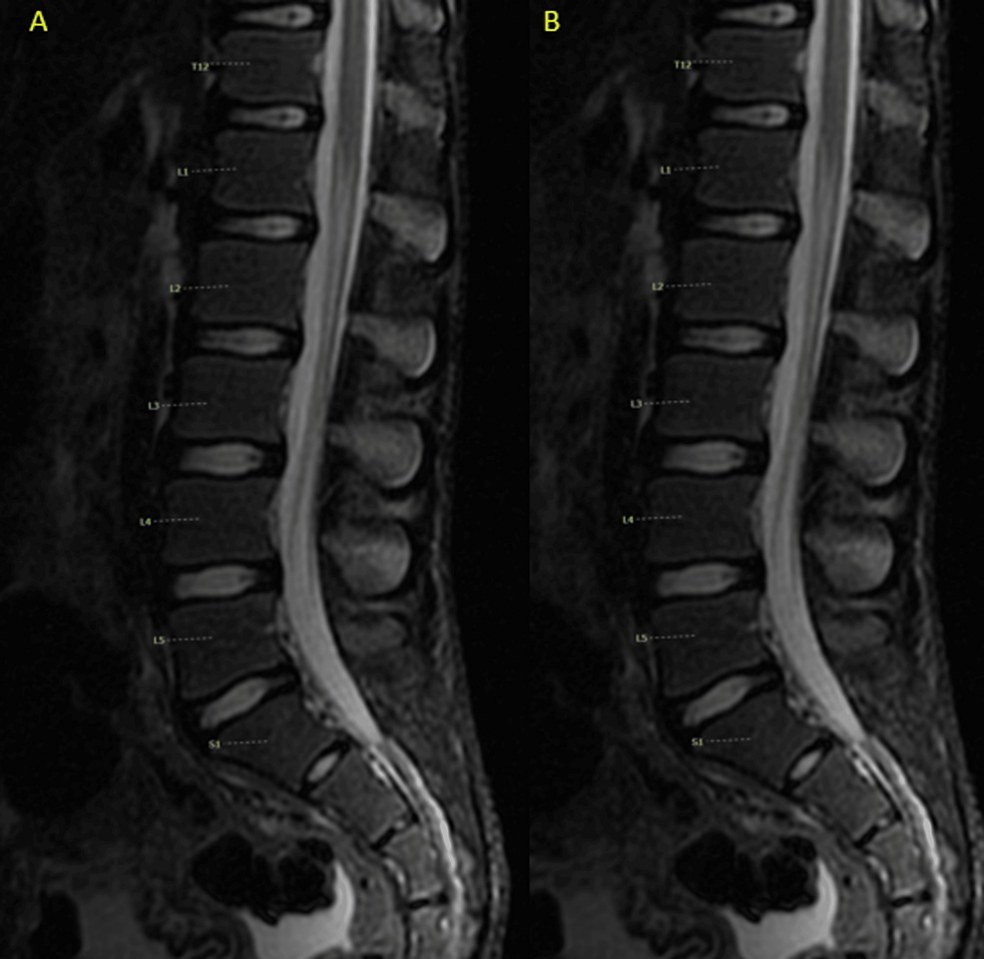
MRI of the lumbar spine T2 (A) and T2 STIR (B) demonstrated a normal-appearing cord and filum, no abnormal signals, and no evidence of disc bulges or spinal canal stenosis.
